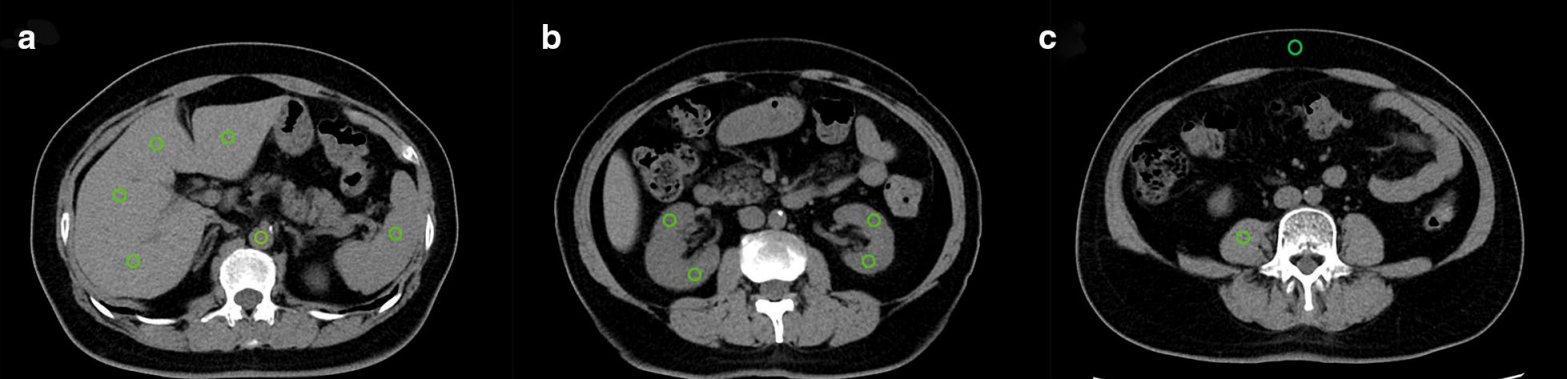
ROIs were placed on the liver, spleen, aorta, kidneys, right psoas muscle, and abdominal-wall subcutaneous fat to measure CT values and noise of various abdominal organs and to evaluate the objective image quality. a shows ROIs located in the liver, spleen, and aorta. b shows ROIs located in the kidneys. c shows ROIs located in the right psoas muscle and anterior abdominal-wall subcutaneous fat
